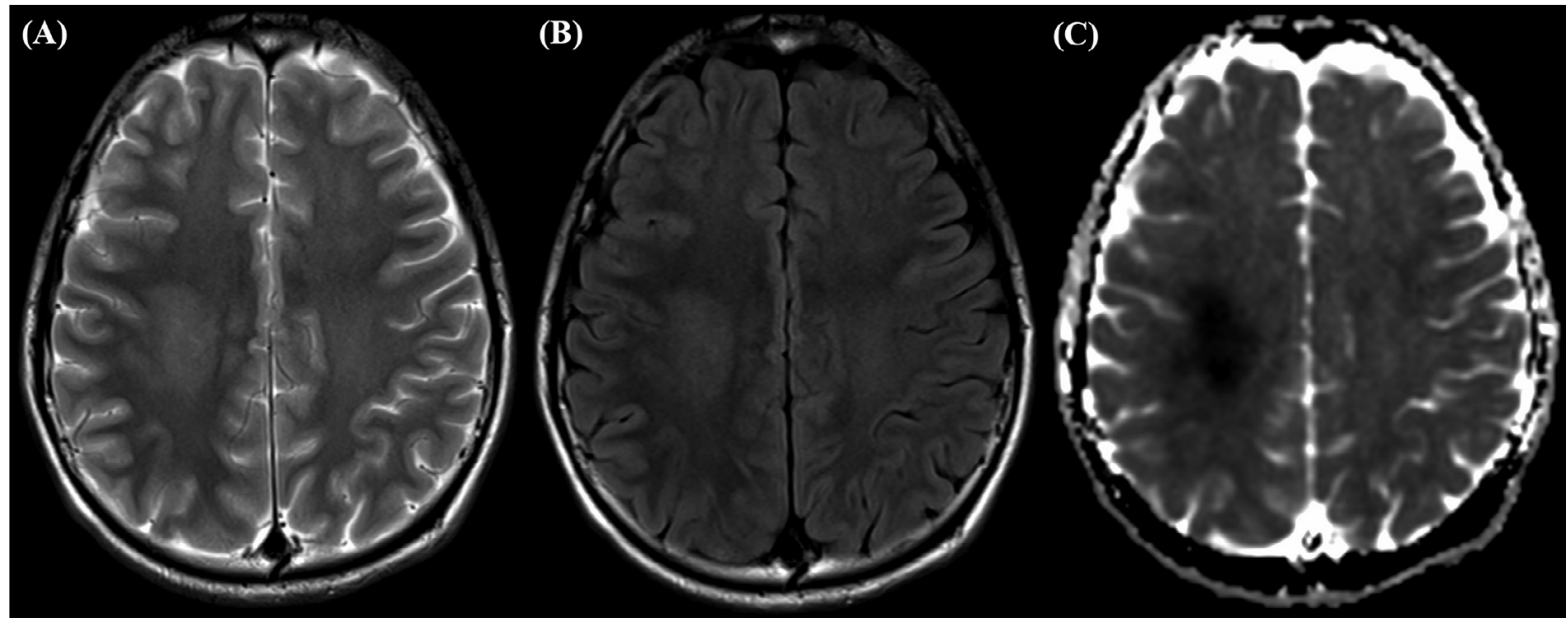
MRI findings taken at the time of presentation, revealing bilateral deep white matter hyperintense foci, greater on the right side, with associated diffusion restriction in the right centrum semiovale on (A) T2-weighted image, (B) FLAIR, (C) DWI.
